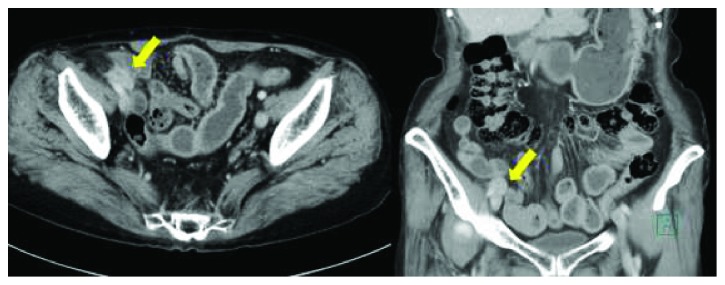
Contrast-enhanced CT scan showing an irregular tumor around the right external iliac vessels and the small intestine, contiguous to the small intestine (arrow).
